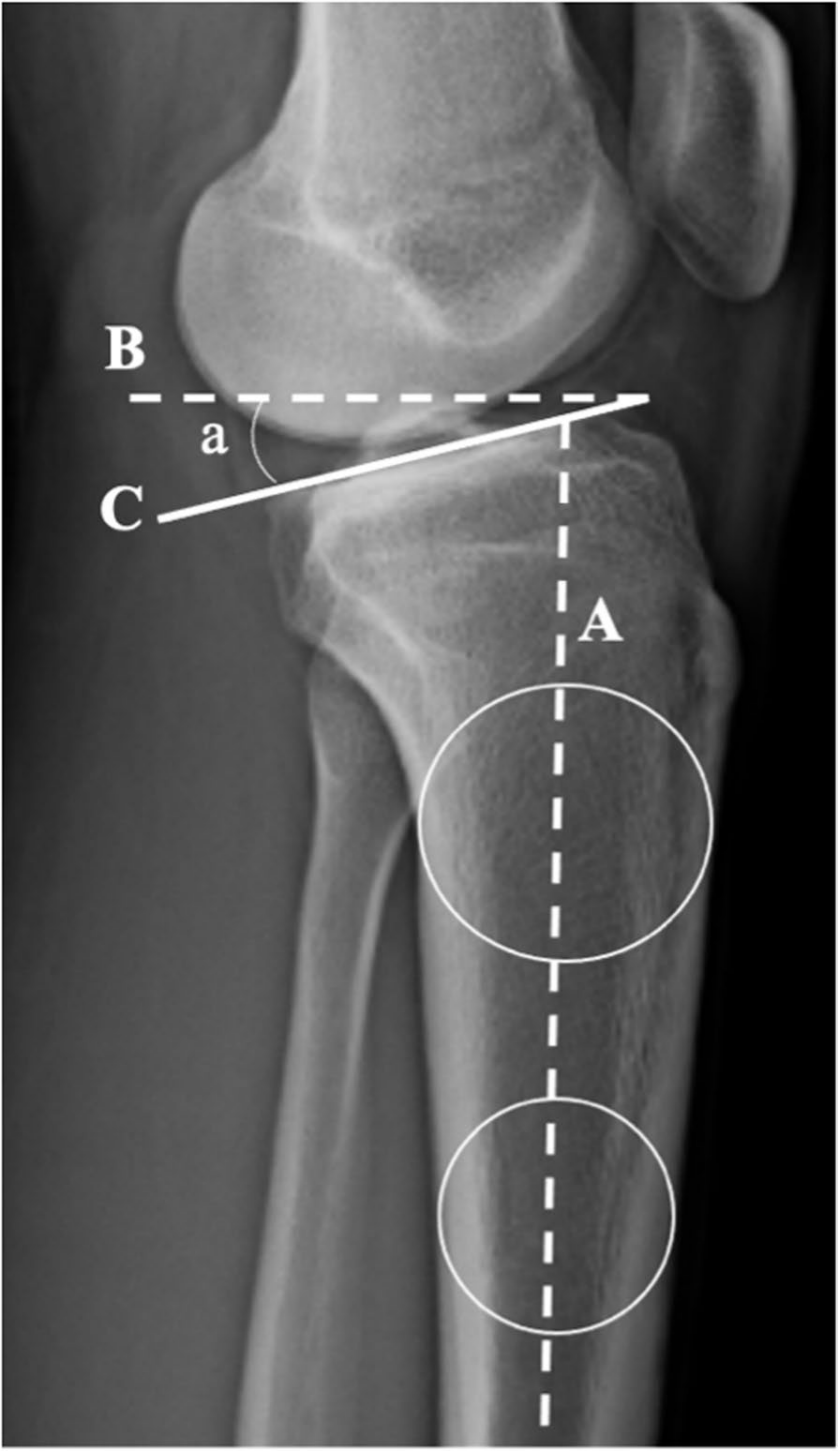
Lateral right knee radiograph demonstrating Posterior Tibial Slope (PTS). Measurement of PTS in monopodal WB x-rays. PTS is the angle formed between a line (B) perpendicular to the tibial diaphyseal axis (A) and the line (C) tangent to the most superior points at the anterior and posterior edges of the medial plateau
